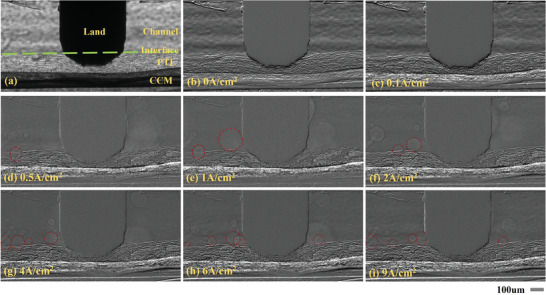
The cell cross‐sectional radiographs of Cf_145 sample a) in the initialization stage with full water and in the constant‐density operation stage for different current densities of b) 0 A cm^−2^, c) 0.1 A cm^−2^, d) 0.5 A cm^−2^, e) 1 A cm^−2^, f) 2 A cm^−2^, g) 4 A cm^−2^, h) 6 A cm^−2^ and i) 9 A cm^−2^ (interfacial separation zones indicated by red lines). S2
